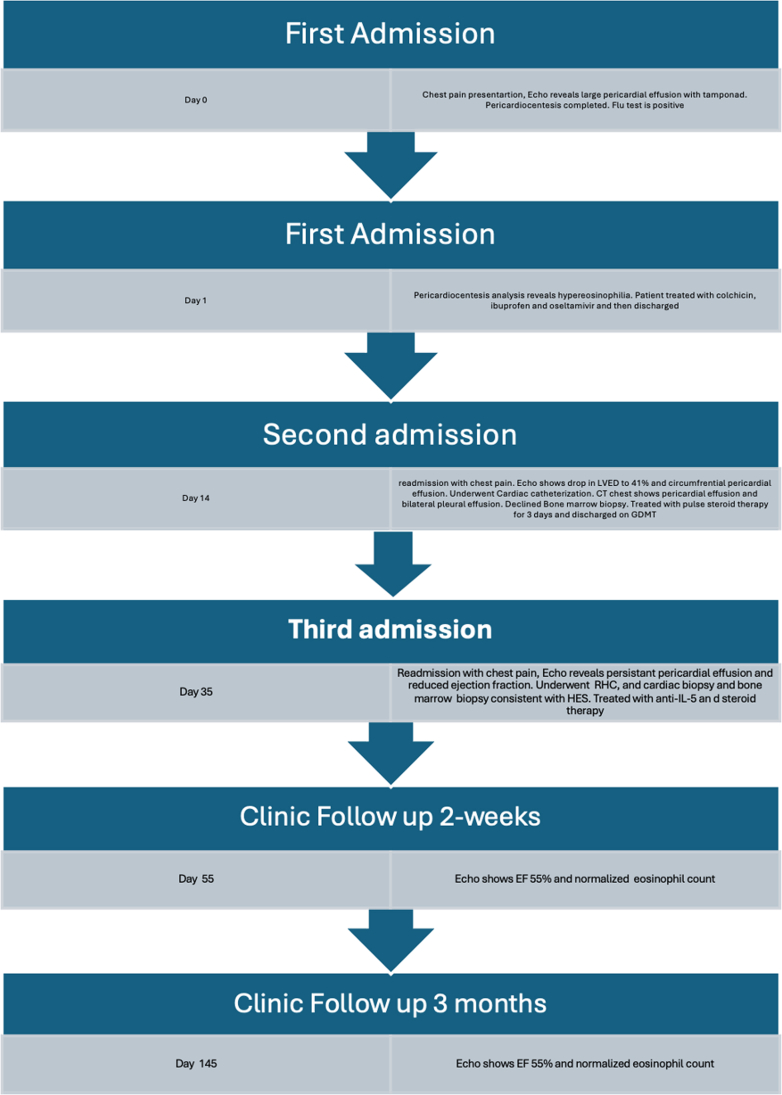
Timeline of Case Presentation and Follow-Up CT = computed tomography; EF = ejection fraction; HES = hypereosinophilic syndrome; GDMT = guideline-directed medical therapy; RHC = right heart catheterization.
